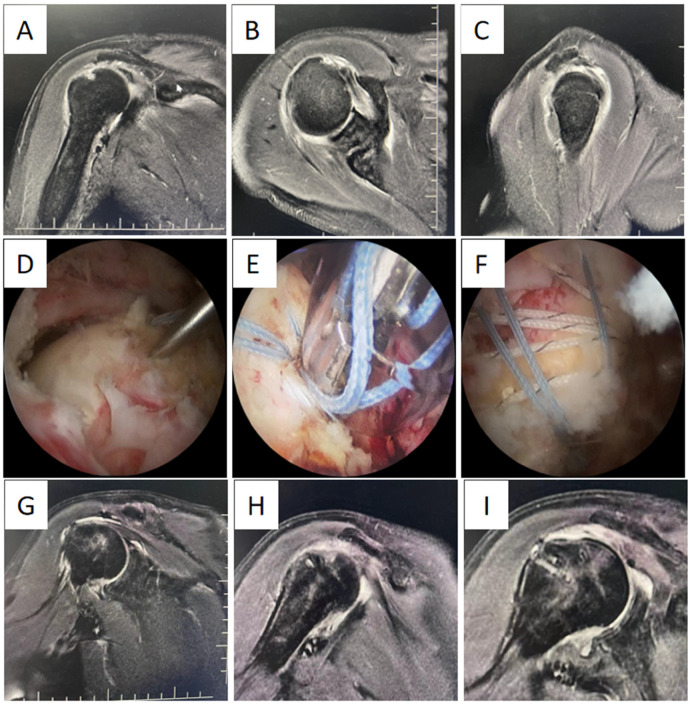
Imaging findings of a failed conventional double-row repair. (A–C) Preoperative coronal MRI showing a full-thickness supraspinatus tendon tear with retraction. (D–F) Intraoperative image showing the torn tendon with poor tissue quality and increased repair tension. (G–I) Postoperative MRI at 3 months demonstrated discontinuity of the repaired tendon with fluid signal evident at the tendon–bone interface, findings consistent with retear (Sugaya type IV–V).
